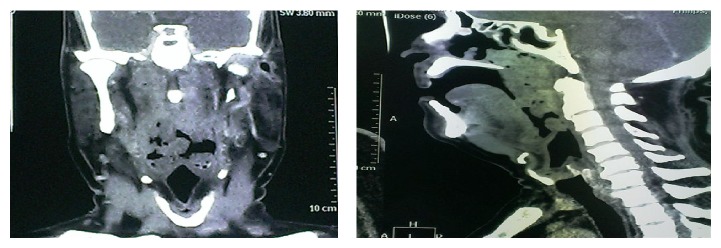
CT scan coronal and sagittal section views showing extension of the tumor in nasopharynx and oropharynx.
